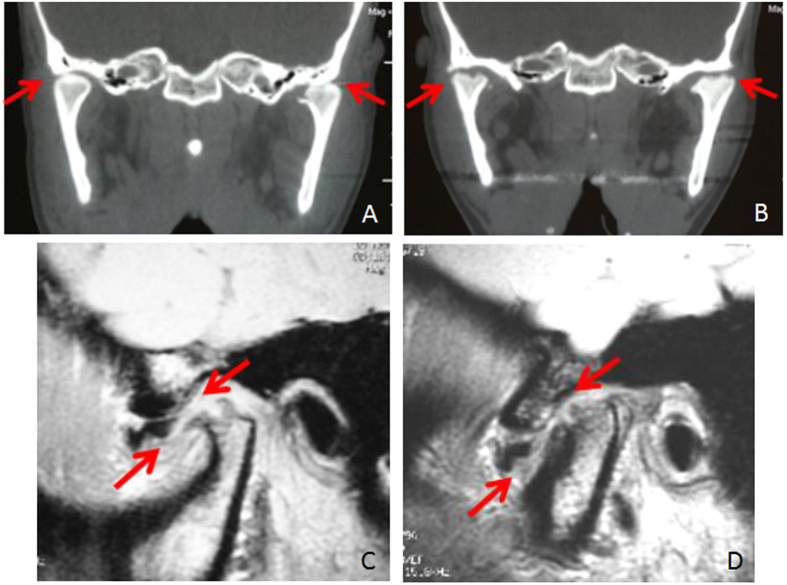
Disc displacement and condylar bone destruction in the end stage. (A) Decreased joint space post injury by coronal CT reconstruction (arrow). (B) Condylar bone resorption 3 months post injury by coronal CT reconstruction. (C) MRI showed right disc displacement with condylar bone destruction (arrow). (D) MRI showed left disc displacement with condylar bone destruction (arrow).
